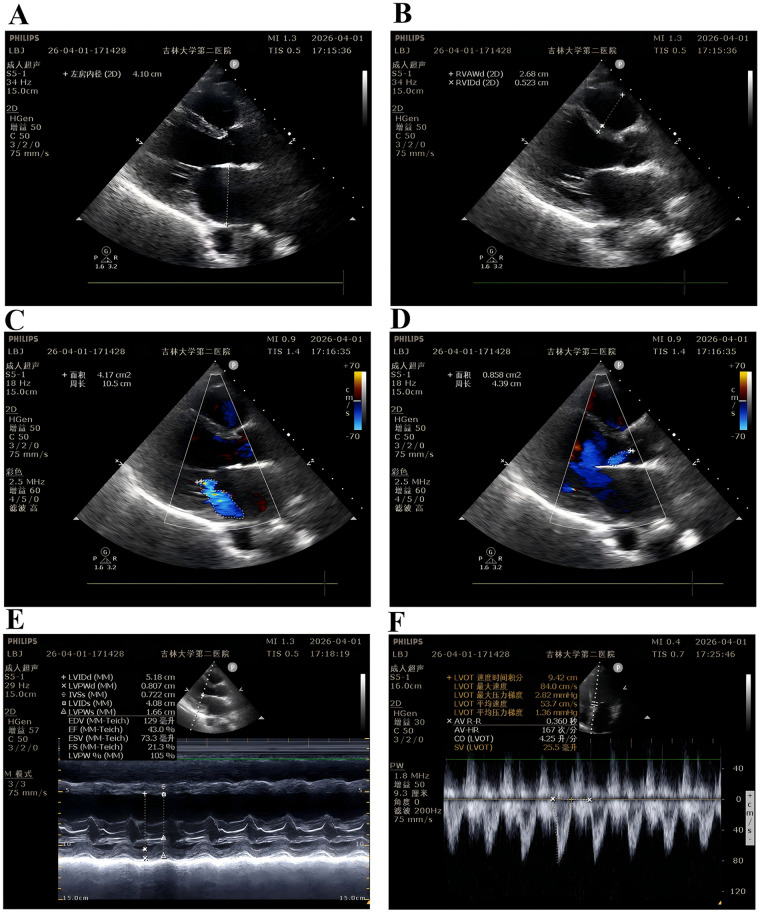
Bedside transthoracic echocardiographic findings before initiation of ECMO support. (A) Parasternal long-axis view showing measurement of the left atrial anteroposterior diameter. (B) Parasternal long-axis view showing measurement of right ventricular anterior wall thickness and right ventricular end-diastolic diameter. (C) Color Doppler imaging showing mitral regurgitation and measurement of the regurgitant jet area. (D) Color Doppler imaging showing aortic regurgitation and measurement of the regurgitant jet area. (E) M-mode echocardiography showing measurement of left ventricular dimensions, with LVEF calculated using the Teichholz method. (F) Pulsed-wave Doppler of the left ventricular outflow tract showing measurement of the velocity-time integral, with calculation of SV and CO.
